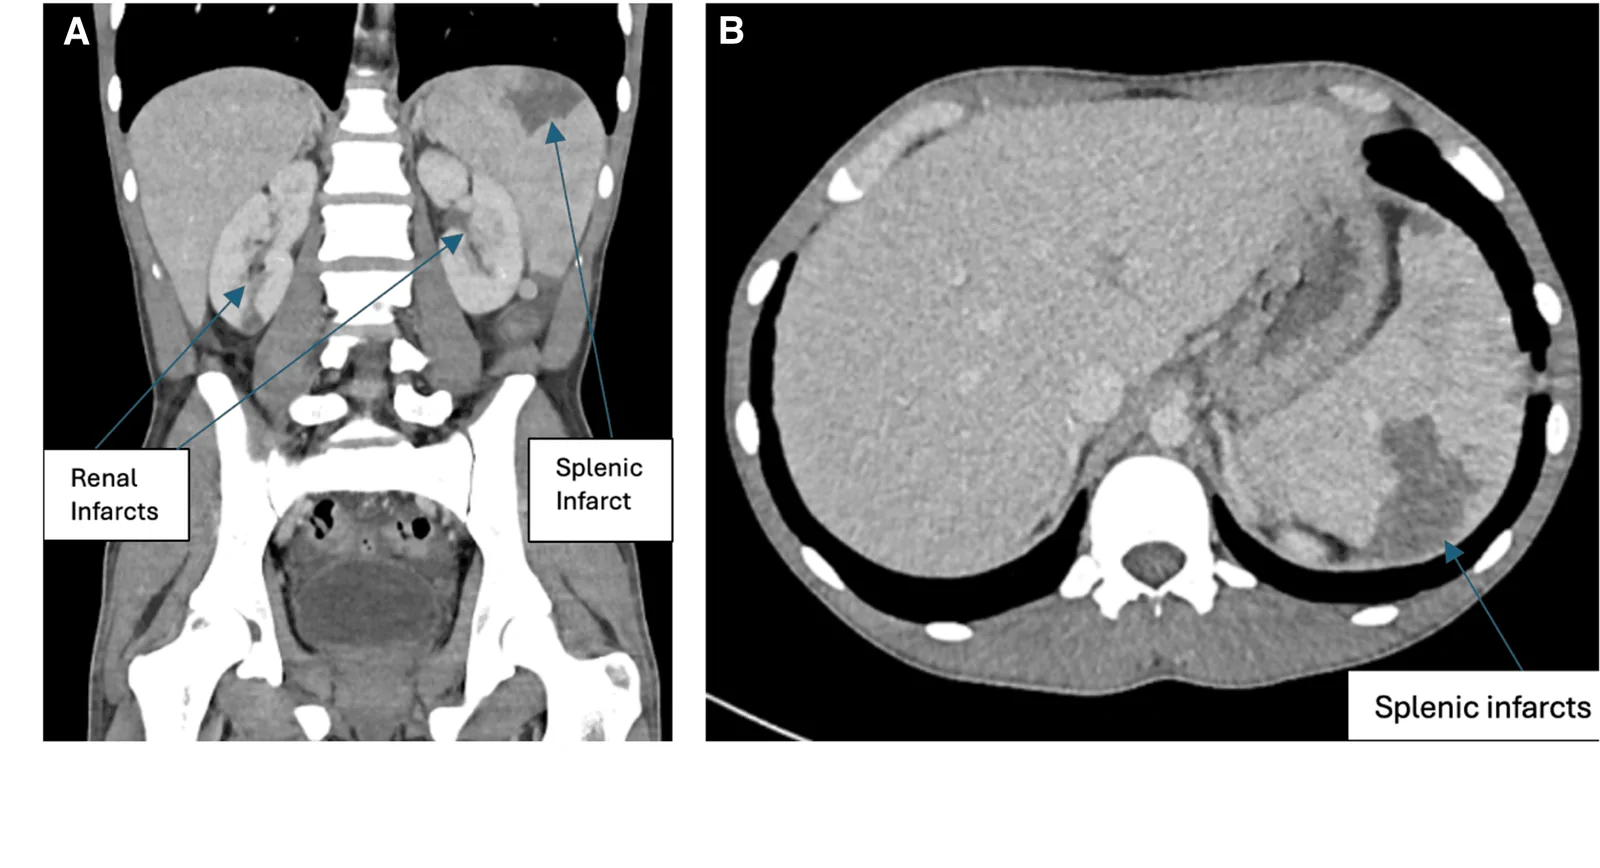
Contrast-enhanced CT of the abdomen and pelvis (A) Coronal view showing bilateral renal infarcts and multifocal splenic infarcts. (B) Axial view showing multifocal splenic infarcts.
